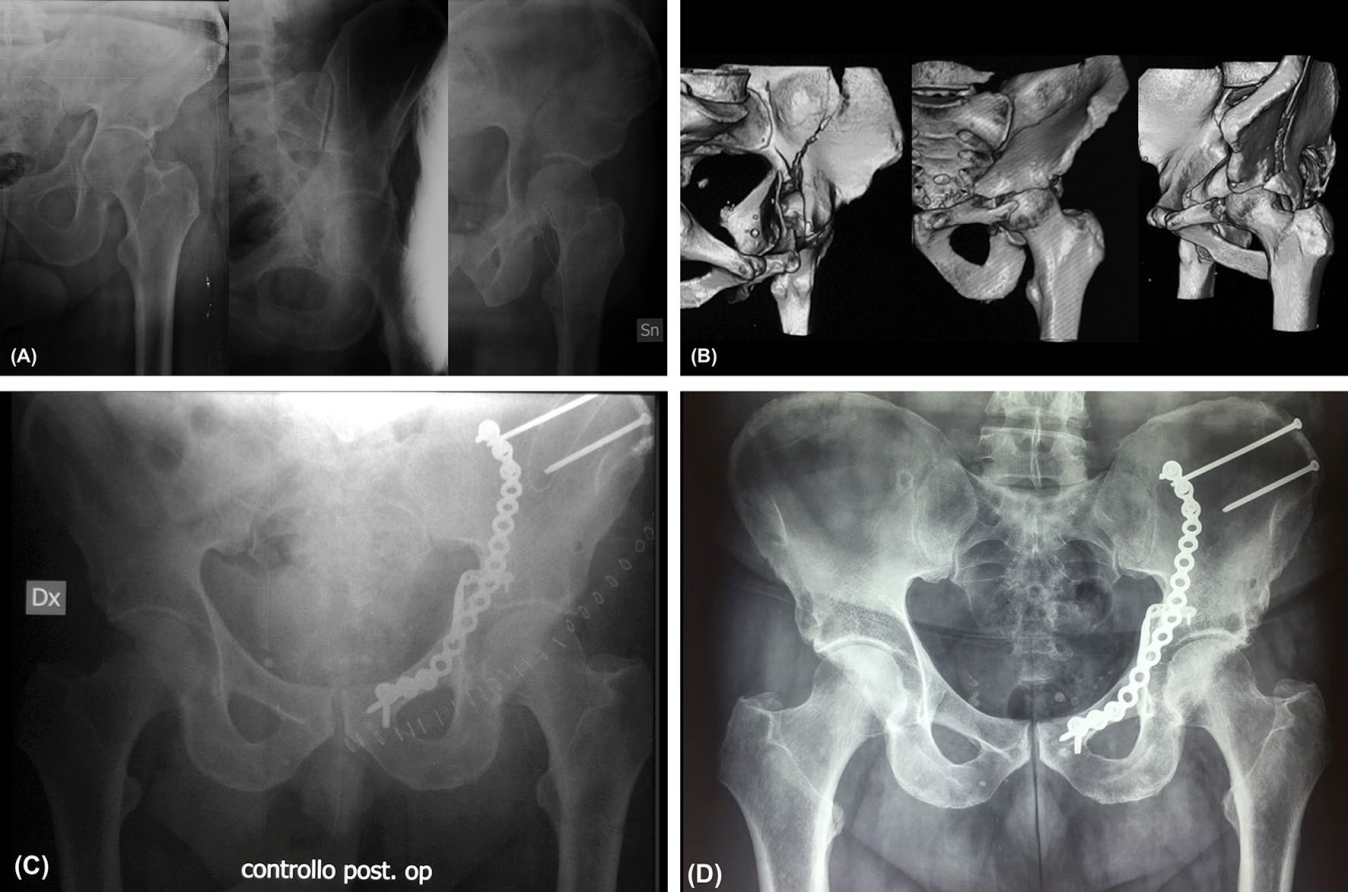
A 61 years old man reported, in a car accident, a left hemitransverse + anterior column acetabular fracture. a Preoperative X-rays: AP view and Judet views. b Preoperative CT. c Postoperative X-rays. d X rays at 24 months follow-up
